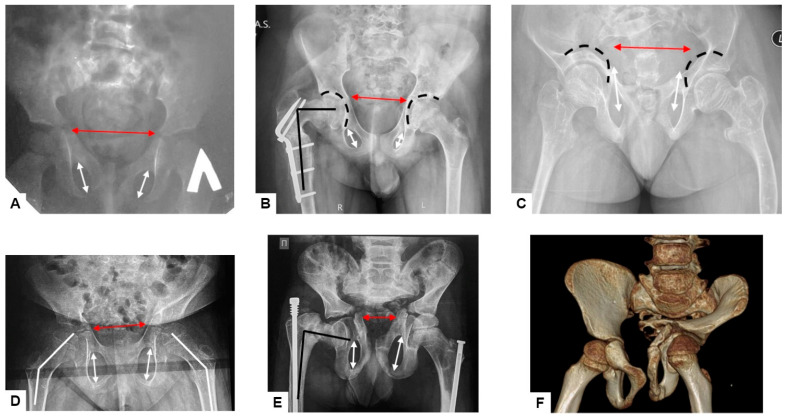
Anteroposterior radiographs of pelvis and hips from patients of the different ages: primary coxa valga (white lines) (D); secondary post-fracture coxa vara (black lines) (B,E); pelvic deformity—narrowing of the anterior part of the pelvic ring (red arrows) (A–E); protrusio acetabuli (black broken lines) (B,C); vertically oriented foramina obturatoriae (white arrows) (A–E). CT scan of the pelvis and hips (F).
